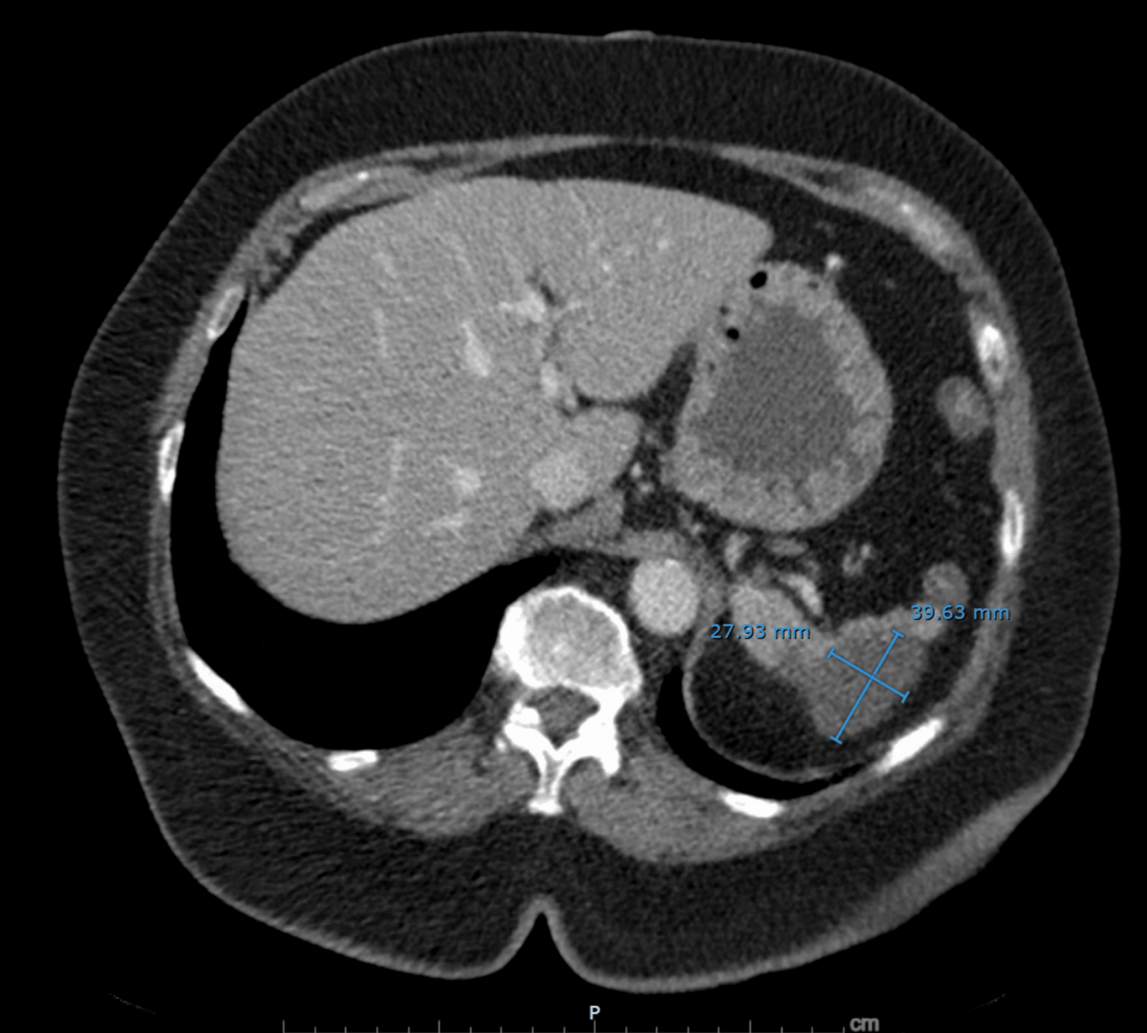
CT demonstrating abnormal splenic enhancement suspicious for splenic infarction. Contrast-enhanced CT of the abdomen demonstrates an atrophic, lobulated spleen with a new 4.0×2.8 cm low-density cystic lesion at the splenic dome and poor opacification of the splenic artery, findings favored to represent splenic infarction. A splenic metastasis was considered less likely.
